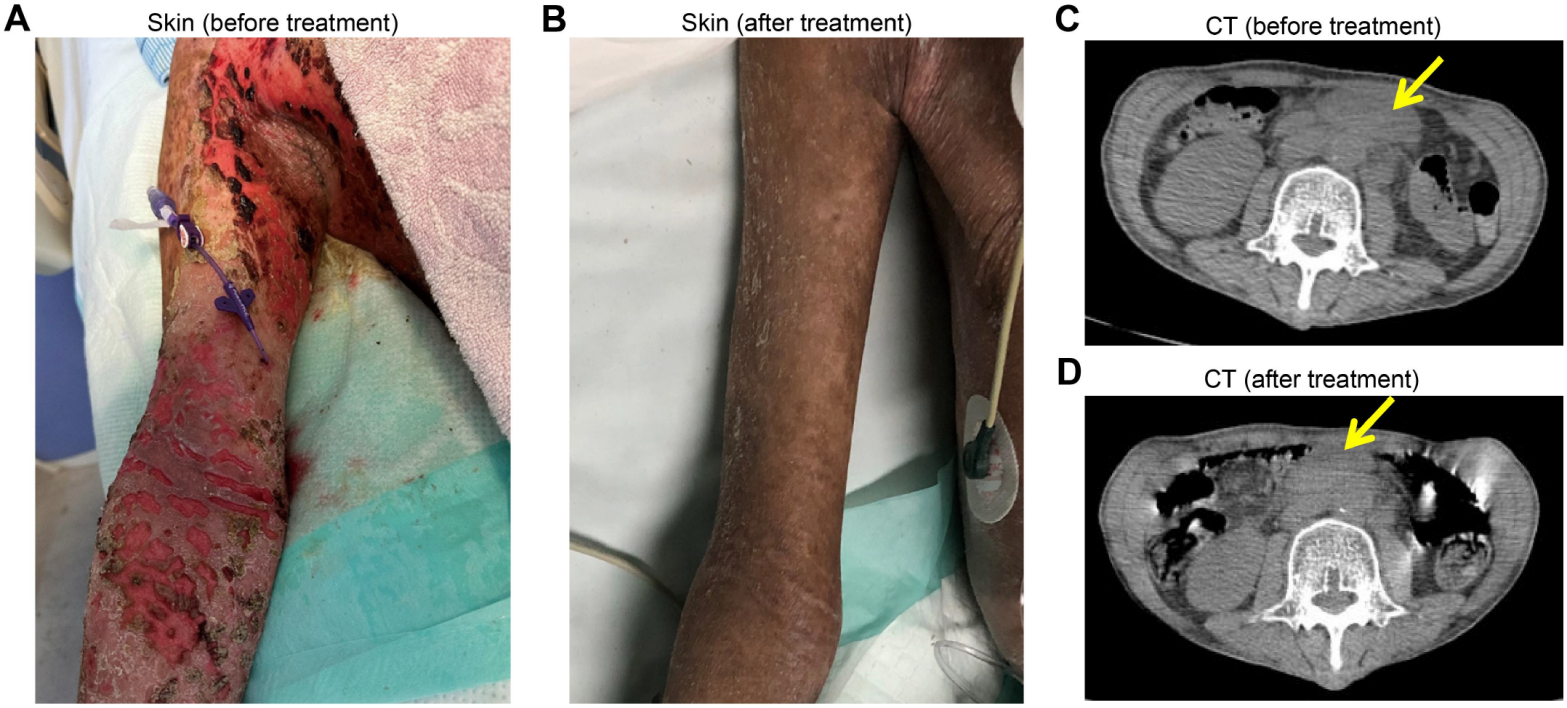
Skin condition and CT scan pre- and post-treatment. (A) Massive cutaneous eruption was noticed in upper extremities before treatment. (B) Skin lesion was improved after treatment. (C) CT scan showed abdominal mass before treatment. (D) CT scan showed reduction of the abdominal mass after treatment.
